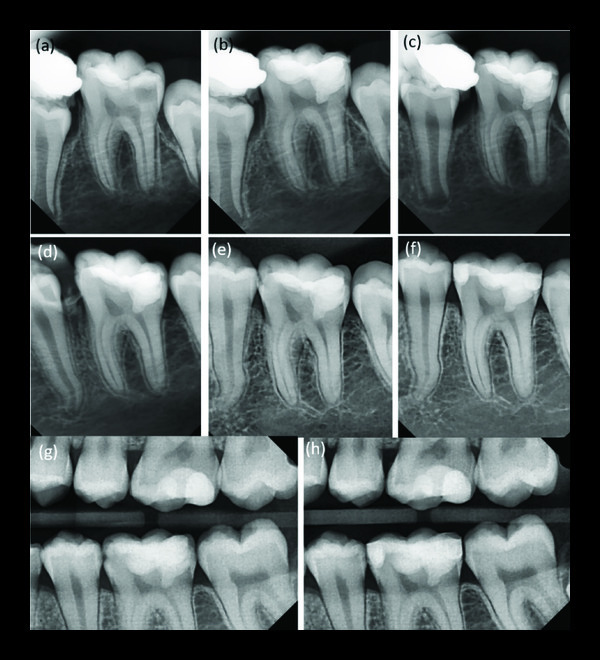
(a) Diagnostic periapical radiograph, (b) after partial pulpotomy, (c) after six months, (d) one-year follow-up showing mineralized bridge formed underneath capping material and normal periapical tissues, (e) 18-month follow-up, (f) two-year follow-up, (g) three-year postoperative bitewing radiograph showing mineralized bridge formed under capping material and new caries at distal and mesial surfaces, and (h) four-year postoperative bitewing radiograph.
